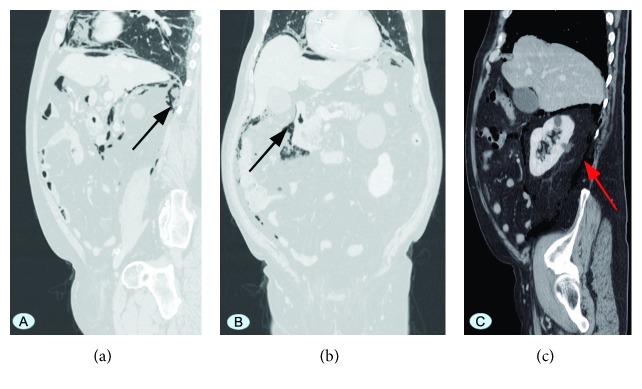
Abdominal CT scan showing pneumoperitoneum and pneumoretroperitoneum (a, b), mainly located at the epimesogastrium, at the right anterior and posterior pararenal and perihepatic spaces (c) (black arrows, red arrow).
